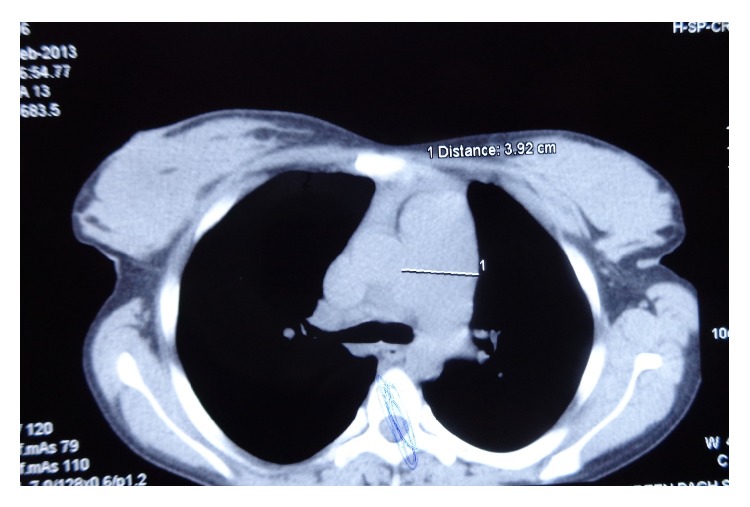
CT chest showing dilated pulmonary artery (marked by 1).
